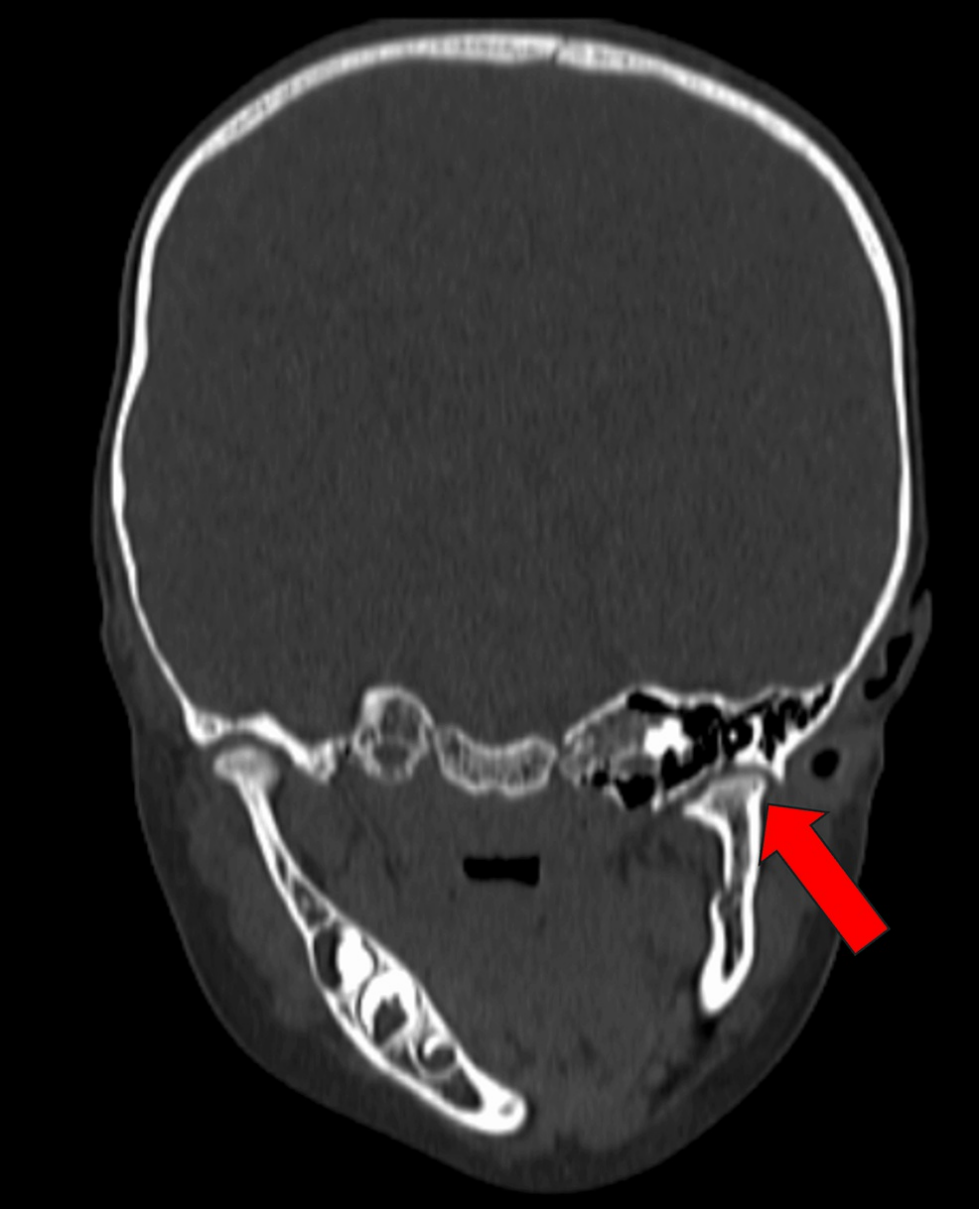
Coronal CT bone window of TMJ showing left mandibular hypoplasia with tilt of the mandible (red arrow). CT: computed tomography; TMJ: temporomandibular joint
